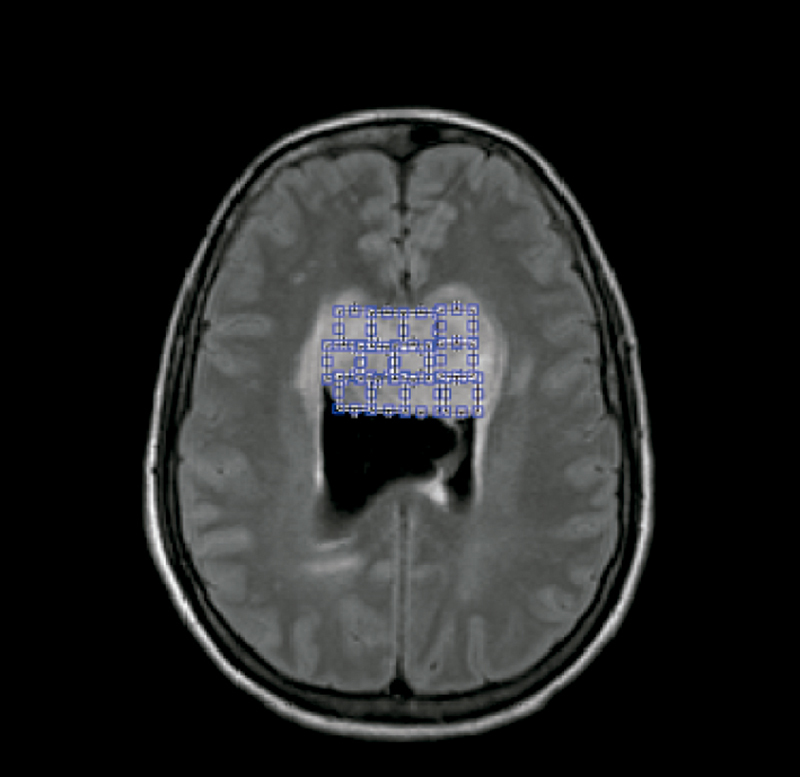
Single slice of a FLAIR weighted image of brain tumor showing the positioning of ROIs within lesions.
